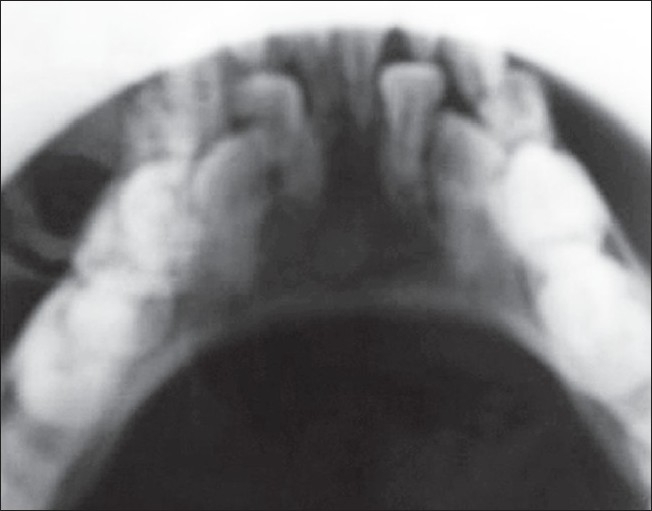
Jaw X-ray showing retention of primary dentition
